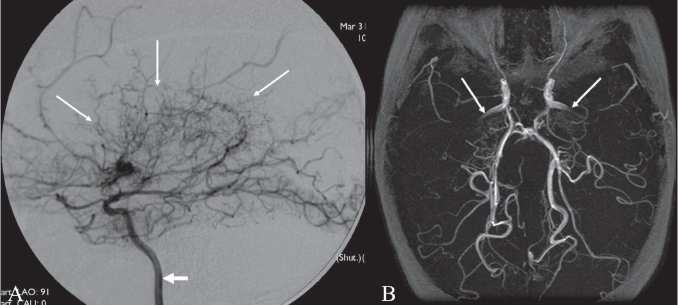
Moya moya appearance. Lateral anterior oblique view (A) of an internal carotid artery (thick short arrow) angiogram shows multiple, small, tortuous collateral vessels in the distribution of the middle cerebral artery (arrows), suggestive of the moya moya (puff of smoke) appearance. Axial view of the MRI angiogram (B) shows complete occlusion of the middle cerebral arteries bilaterally. Arrows indicate the internal carotid arteries
